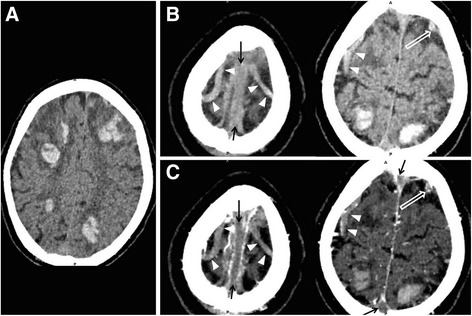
Typical images of the concomitant cortical vein thrombosis on CT and CT venography in a 61-year-old male patient with cerebral venous sinus thrombosis. Non-enhanced CT reveals multiple hemorrhages in bilateral frontal and parietal lobes (a). Cord-like (b, white arrowheads) and dot-like (b, hollow white arrow) hyperdensity (representing acute thrombosis) in the sites of cortical veins can be observed in non-enhanced CT. Non-enhanced CT also reveals the cord-like hyperdensity in the site of superior sagittal sinus (b, black arrows). On the source images of CT venography (c), filling defects of contrast agent can be detected in the sites of cortical veins (white arrowheads and hollow white arrow) and superior sagittal sinus (black arrows)
